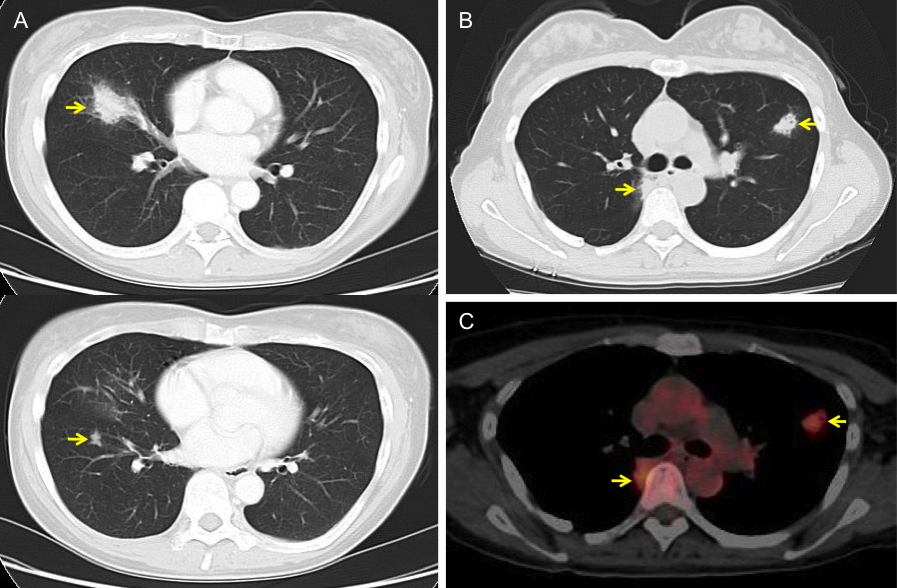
CT and PET/CT performed before the first (A) and second (B, C) surgery.  A, A 30-mm infiltrative lesion and a 6-mm nodule are seen in the right S4 and S8, respectively. B, An 18-mm nodular lesion and a 16-mm infiltrative lesion are seen in the left S3 and right S2, respectively. C, PET/CT shows fluorodeoxyglucose uptake within these lesions. The arrows indicate the lesions.
